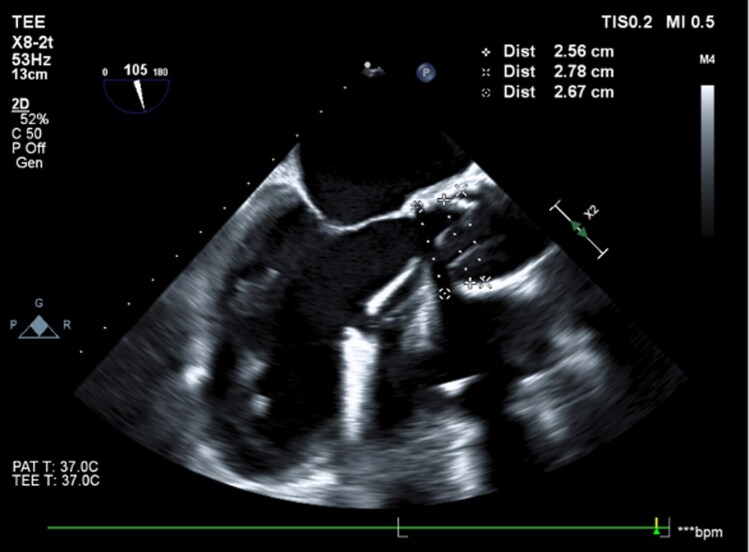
A transoesophageal echocardiogram image showing valve sizing on preprocedural transoesophageal echocardiogram.
